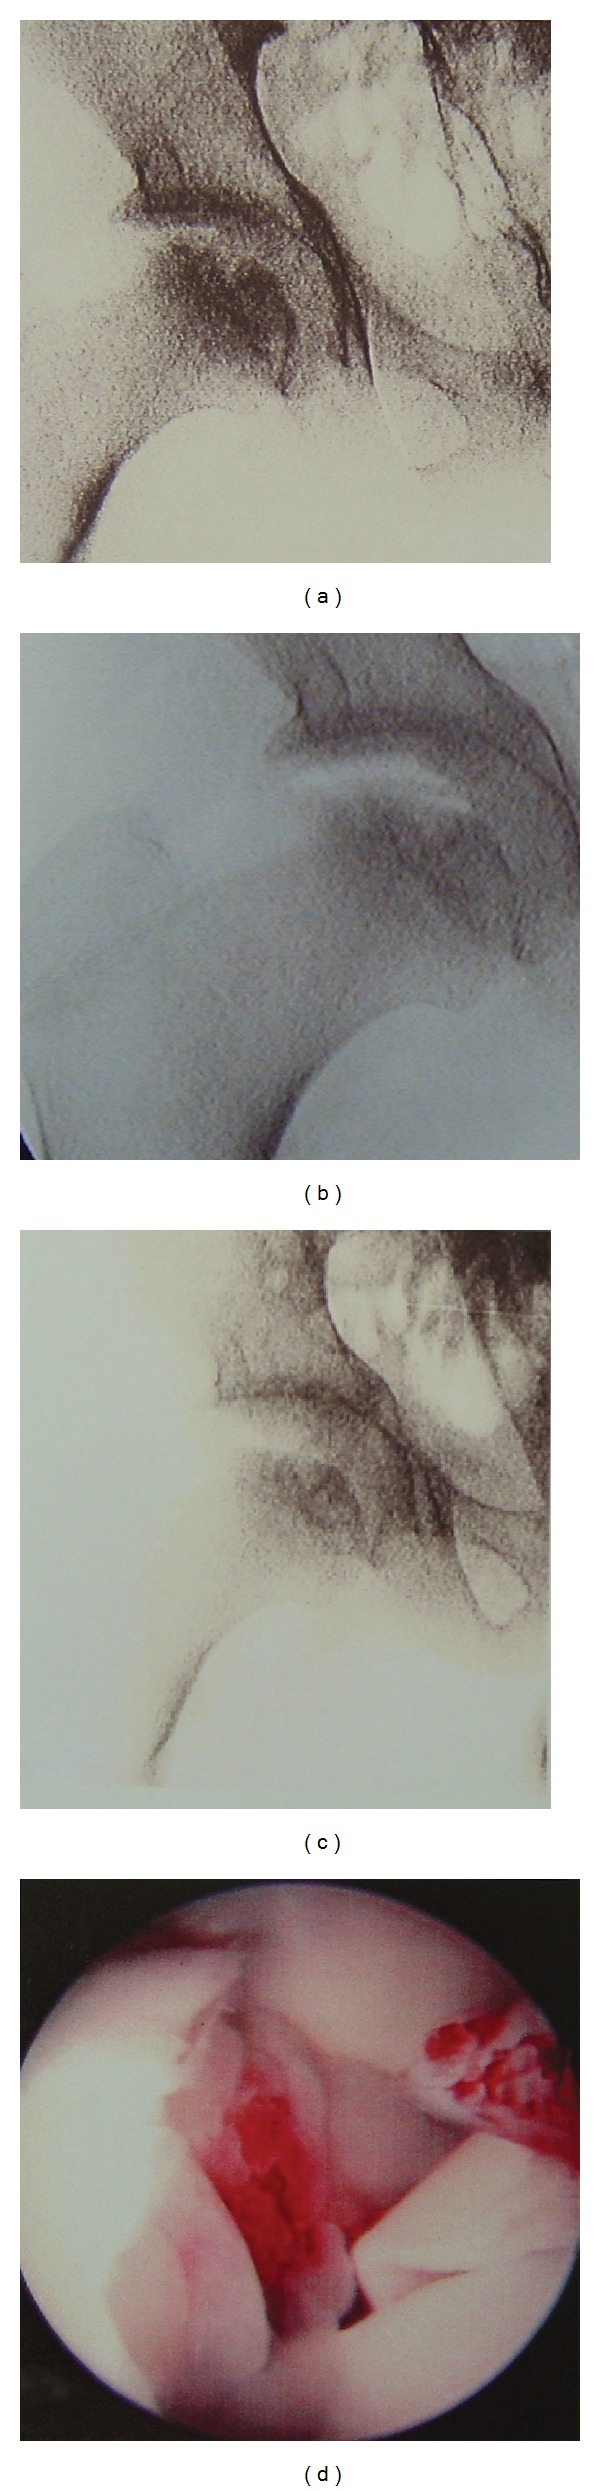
(a) Fluoroscopy of the right hip of a 15-year-old boy with Perthes disease prior to traction. (b) One minute of traction: the vacuum phenomenon shows a “double bubble” effect—a dynamic detachment of cartilage from the femoral head that was not evident on MRI. Vacuum fails to develop in the medial half of the joint. (c) Eight minutes of traction: the hip was further distracted and the vacuum phenomenon disappeared. (d) Arthroscopic view of the right hip shows chondrolysis of the femoral head. Massive synovial proliferation obliterates the medial aspect of the joint.
